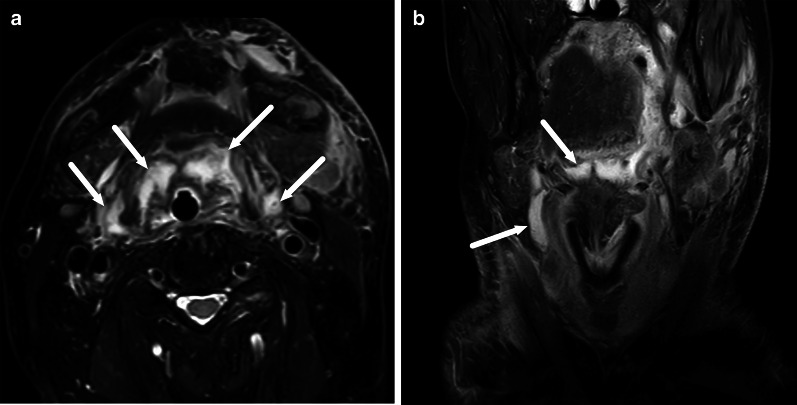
A case of fatal necrotizing fasciitis following odontogenic infection. Axial (a) and coronal (b) fat-suppressed T2-weighted images show extensive soft tissue edema, fluid along fascial planes, and multi-space abscesses (arrows)
